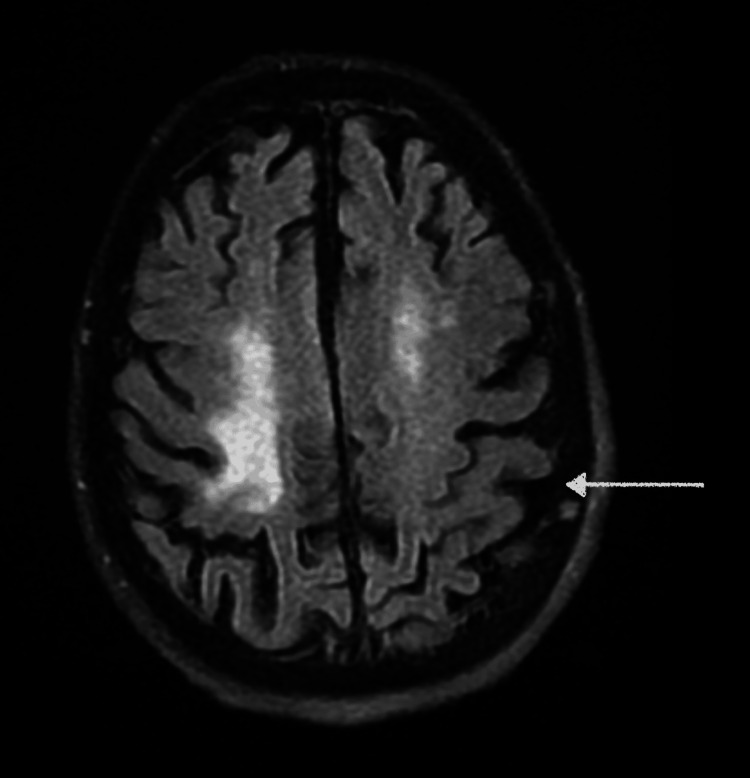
MRI brain revealing diffuse cerebral volume loss with no acute findings (arrow) MRI: magnetic resonance imaging
